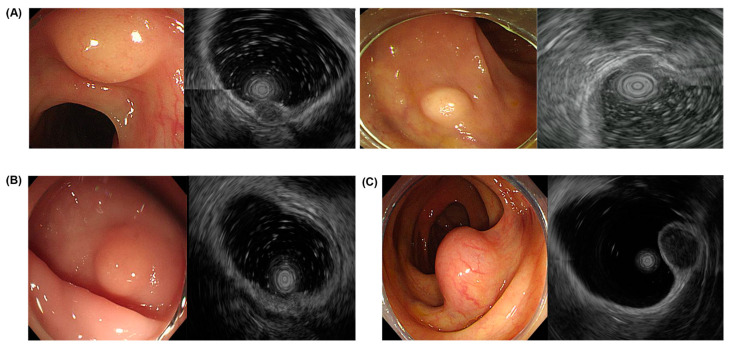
EUS findings of potentially malignant lesions. (A) Granular cell tumor, (B) neuroendocrine tumor, and (C) perivascular epithelioid cell tumor. EUS, endoscopic ultrasound.
